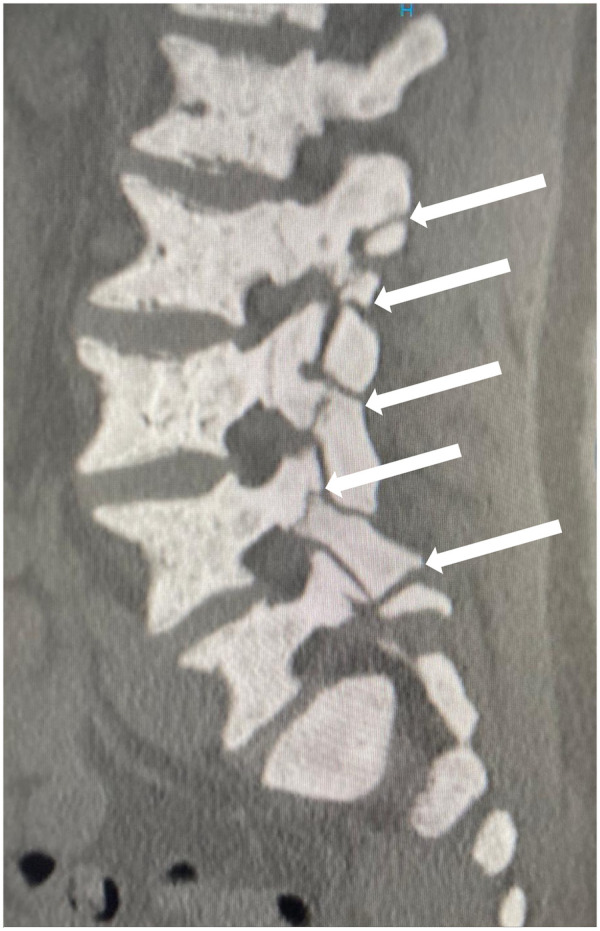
Midline sagittal CT scan image of the lumbar spine showing pars interarticularis defects (white arrows) at multiple levels of the lumbar spine.
